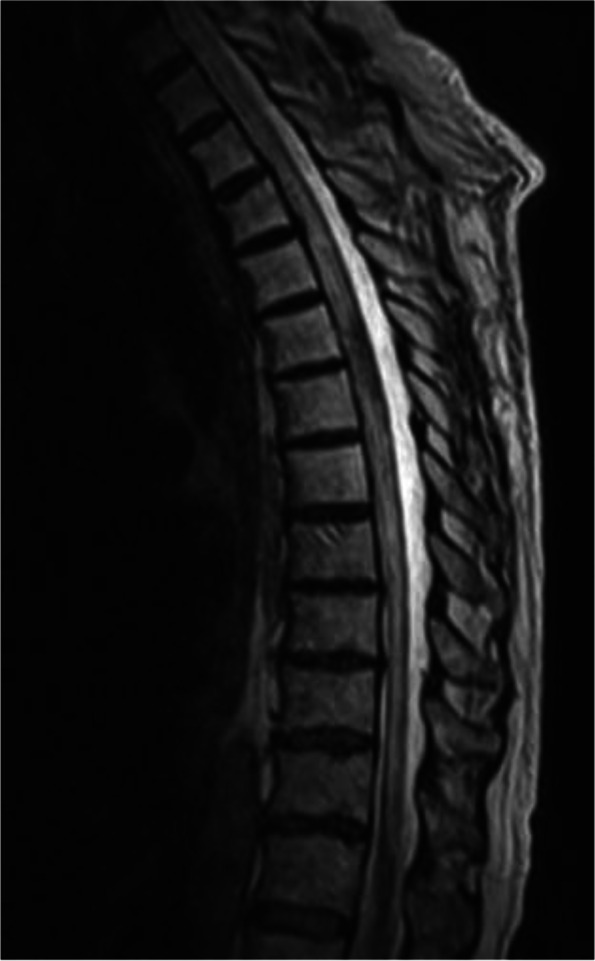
MRI thoracic spine; Sagittal T2. Showed extensive T2 hyper intense signal (C5 till T11)
